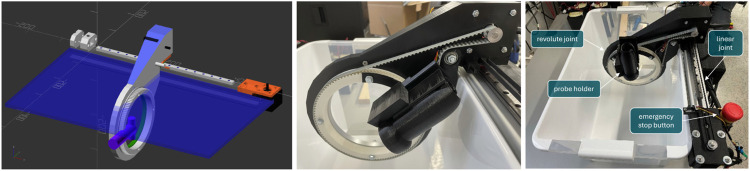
Construction of the prototype. Left: CAD model of the robotic parts, centre: details showing both belt drives and the ultrasound probe holder, right: complete prototype.
